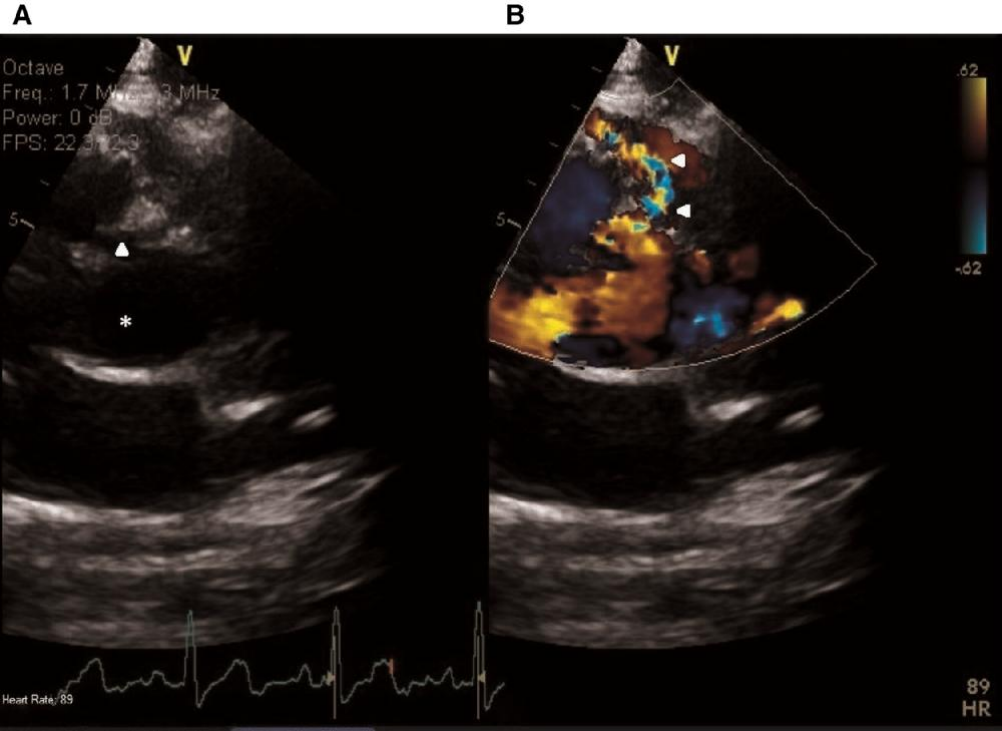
Initial transthoracic echocardiography. Two-dimensional parasternal long-axis, simultaneous colour Doppler view. (*A*) The aortic root (asterisk) and the prominent origin of the right coronary artery (arrowhead) are depicted. (*B*) Colour Doppler imaging showed turbulent flow, probably down to the right coronary artery (arrowheads).
